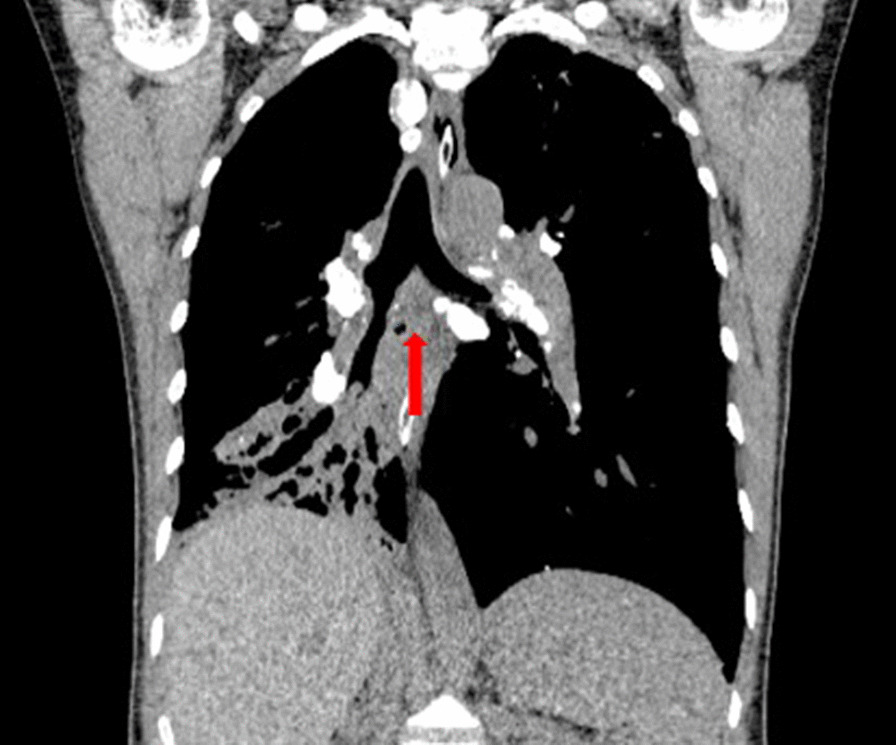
Chest CT scans shows no residual broncholith in the previous location (red arrow) (patient 11, after five times procedures)
